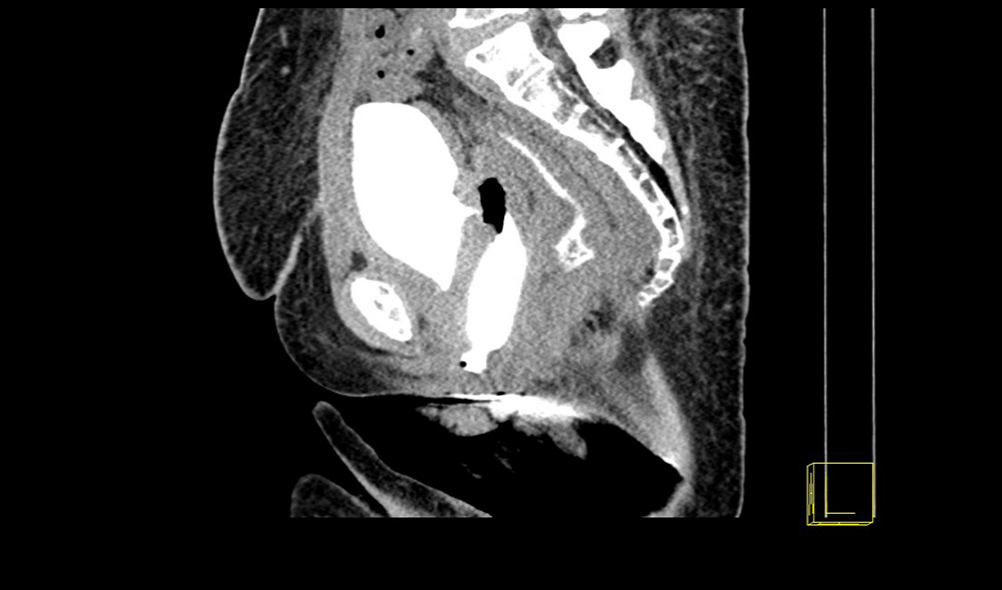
Delayed post-intravenous contrast CT image (sagittal reformat) showing the opacification of the vaginal tampon and the presence of a fistulous tract between the posterior bladder wall and the vagina for case 1.
